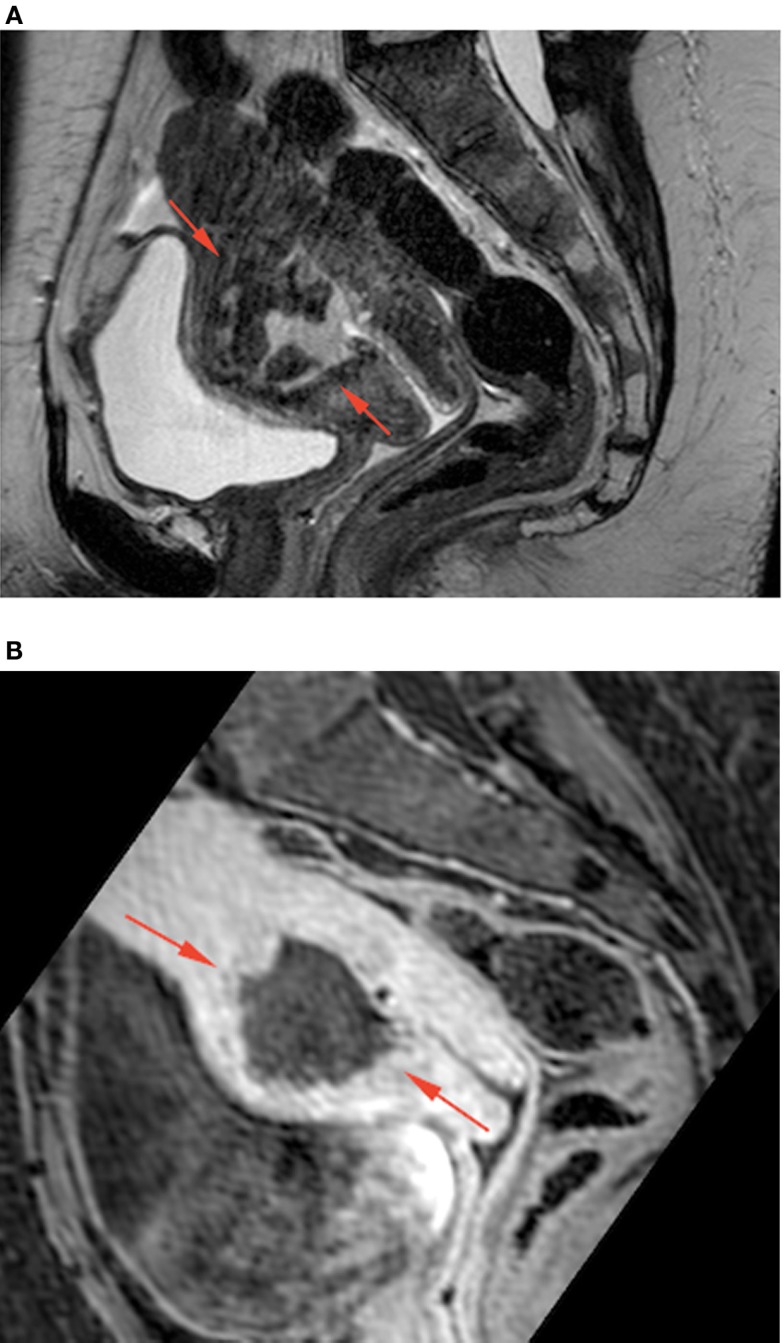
Sagittal T2-weighted TSE (A) and Dixon T1-weighted fat saturated after iv gadolinium injection (B) MRI images at presentation, showing a cavity with thick enhancing walls (red arrows) containing heterogeneous fluid and solid non-enhancing material at the anterior wall of the uterus at the site of cesarian section.
